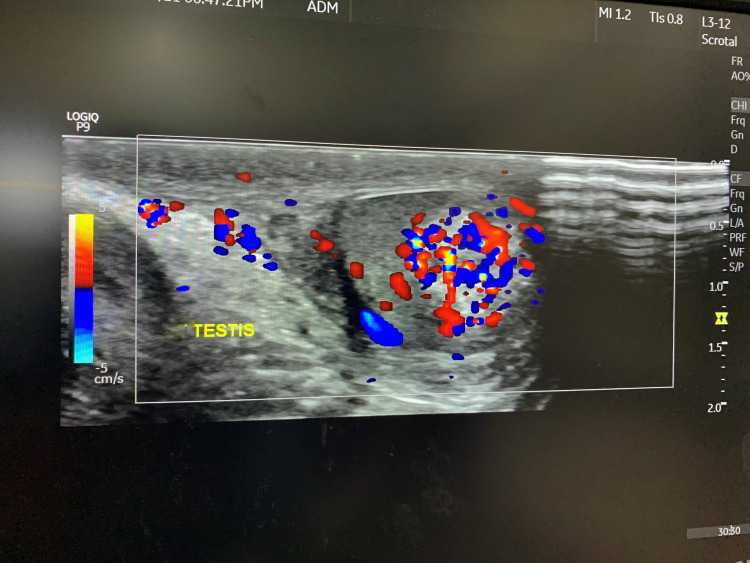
Hypervascular left testes mass on Doppler study.
